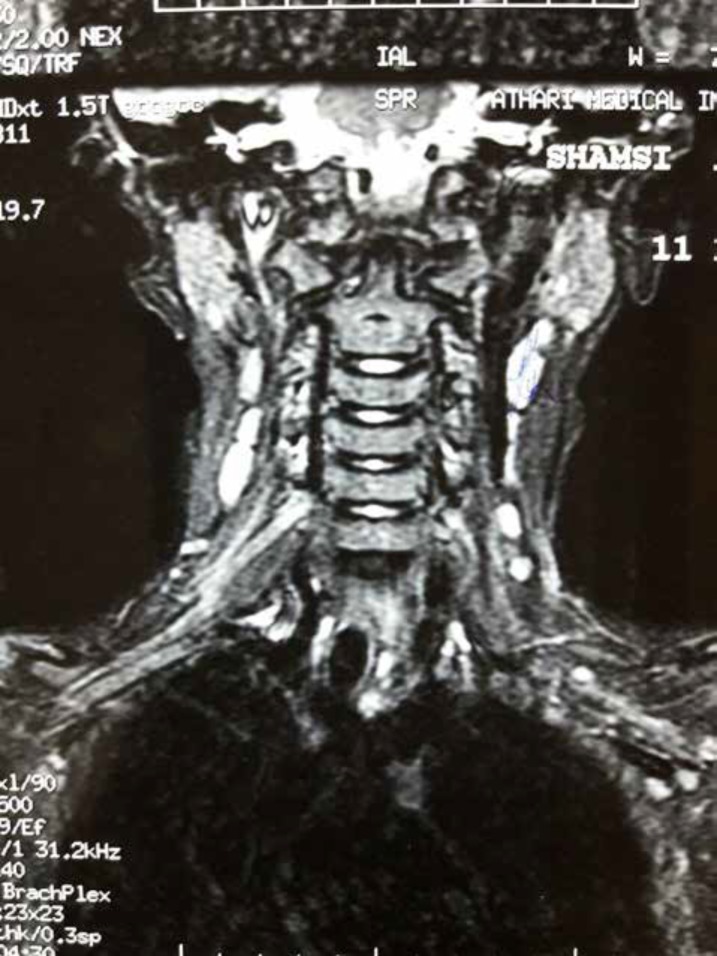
Cervical MRI showing root hypertrophy and enhancement in the right side (C4-C8), with more hypertrophy and enhancement at C4-C6 root
